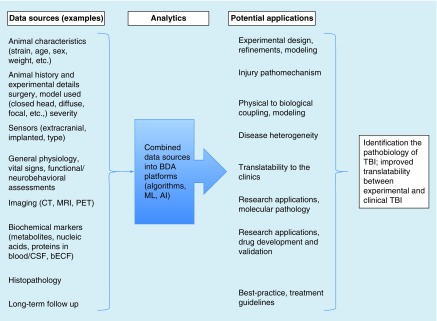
Overview of potential Big Data Analytics approaches in experimental traumatic brain injury. Examples of data sources (see also [42]) and potential application using BDA approaches that can improve modeling, understanding the pathobiology and translatability between experimental and clinical TBI. AI: Artificial Intelligence; BDA: Big Data Analytics; bECF: Brain extracellular fluid; CSF: Cerebrospinal fluid; CT: Computer-assisted tomography; ML: Machine Learning; TBI: Traumatic brain injury.
